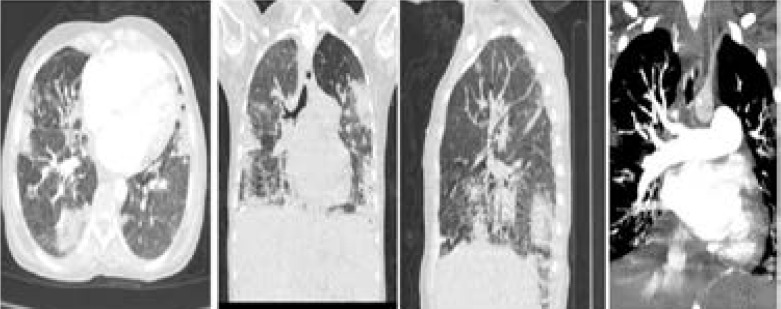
Follow-up Axial, coronal and sagittal planes of thin-section non-contrast CT image in pulmonary parenchymal taken 6 days after (From 8/4/2020), show increased density of GGOs which then progressed into consolidations with perilobular thickening. Chest CT with contrast at coronal plane in mediastinal-window, show neither pulmonary embolism.
